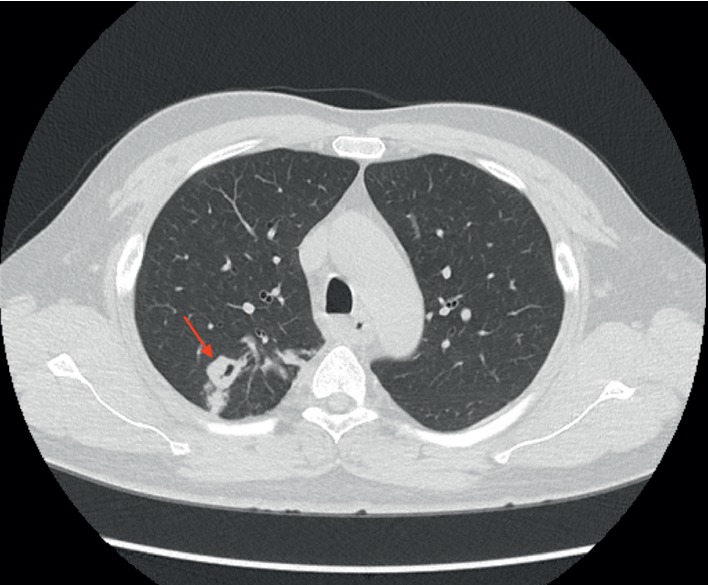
Chest CT scan revealing a new thick-walled cavitary nodule in the right upper lobe (red arrow).
